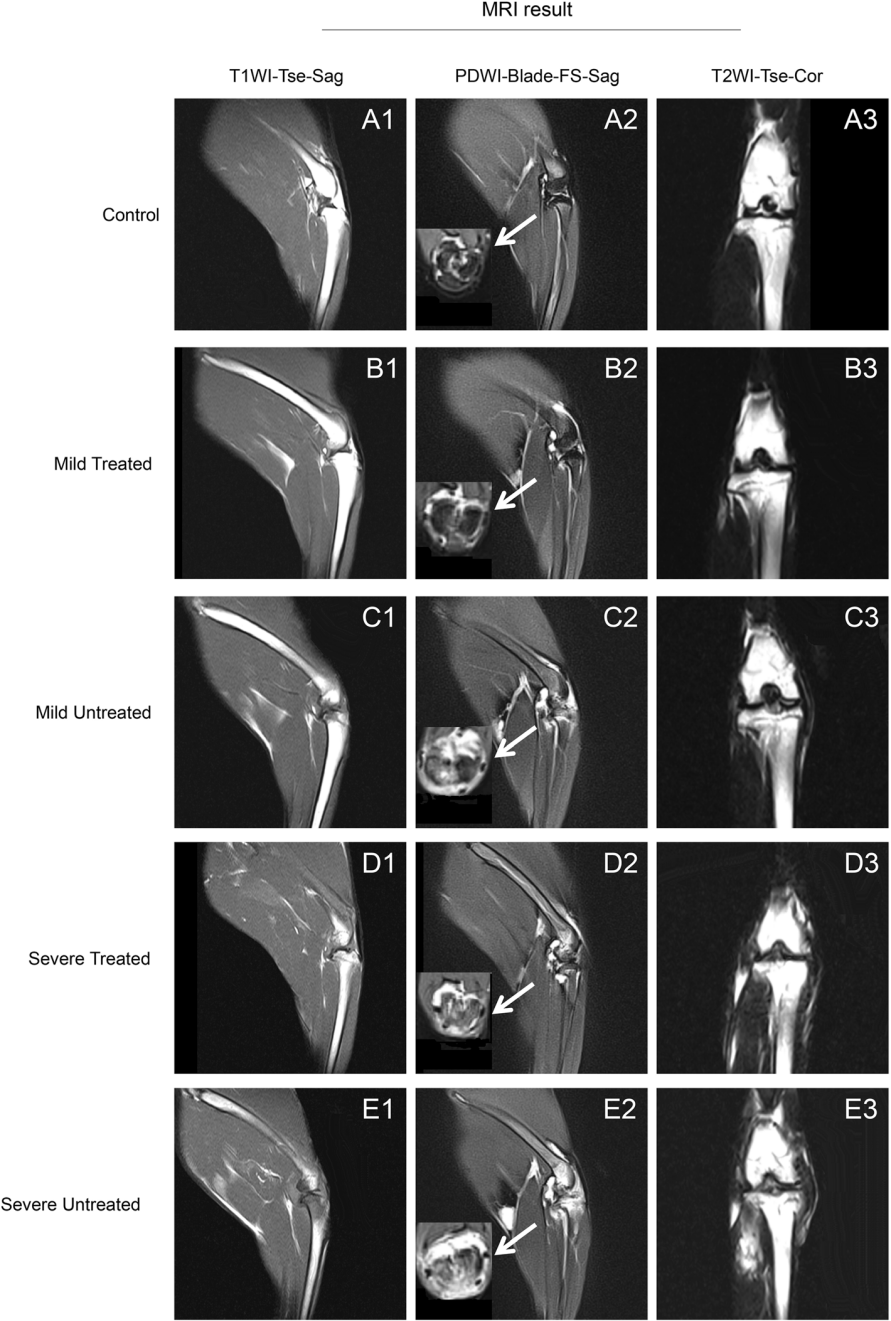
Representative MRI images for imaging diagnosis. T1WI sequence (A1, B1, C1, D1, E1) from the sagittal plane and PDWI-FS (A2, B2, C2, D2, E2) sequence from the sagittal plane and cross plane. T2WI sequence (A3, B3, C3, D3, E3) from the coronal plane
